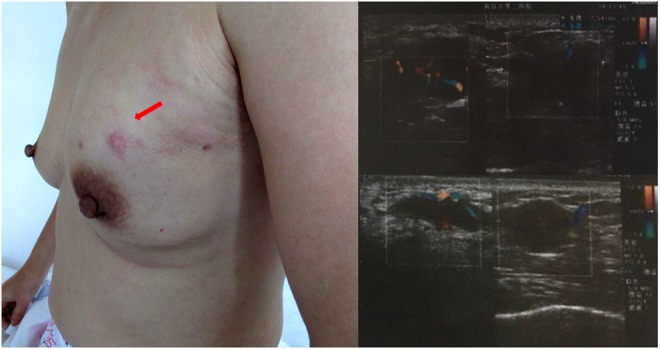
Initial clinical manifestation of the patient A red nodule located in the left upper side of left breast and several palpable lymph nodes in the homolateral axillary fossa region. Diagnostic ultrasound demonstrates hypoechoic mass and suspicious left axillary lymph nodes at initial presentation (pre-biopsy).
